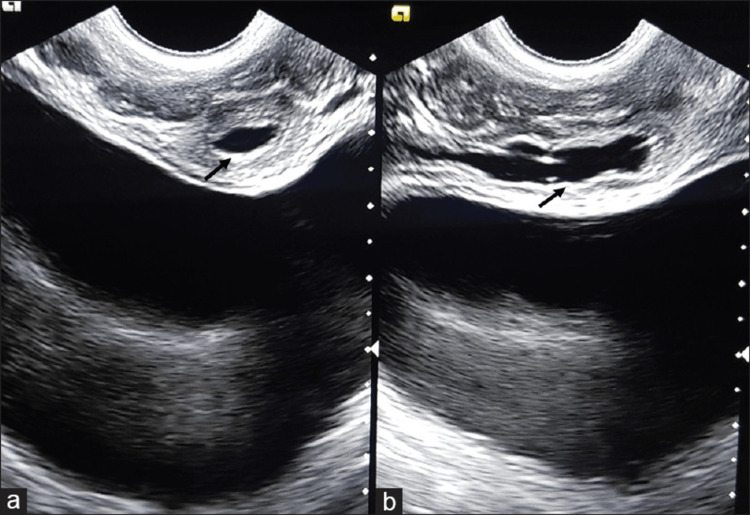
(A, B) Trans-rectal ultrasound image showing cystic structure in the seminal vesicle region.
